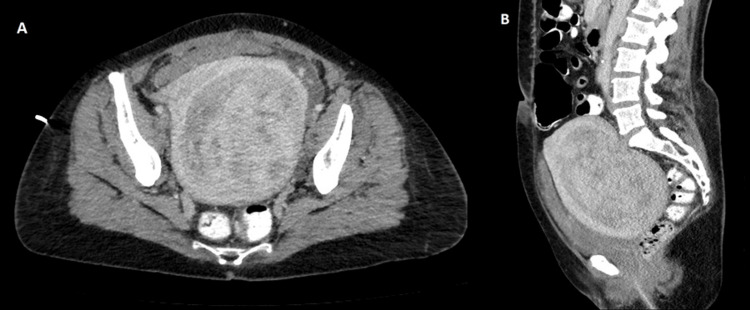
Computed tomography (CT) scan with intravascular contrast confirmed a large heterogeneously enhancing uterine mass, which may be intramural or endometrial in origin, seen in cross-sectional and sagittal views in pictures A and B respectively.
